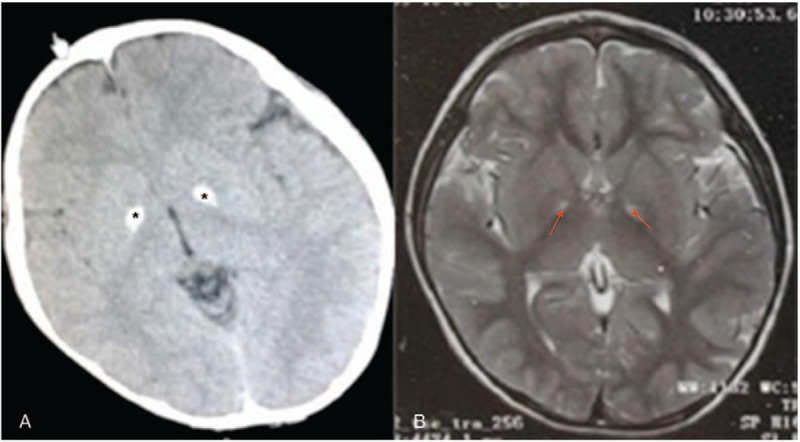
Brain CT scan displayed a bilateral calcification of the globus pallidus (A, black star) and axial T2 weighted brain MRI displaying typical “eye of the tiger” (B, red arrow). CT = computed tomography; MRI = magnetic resonance imaging.
